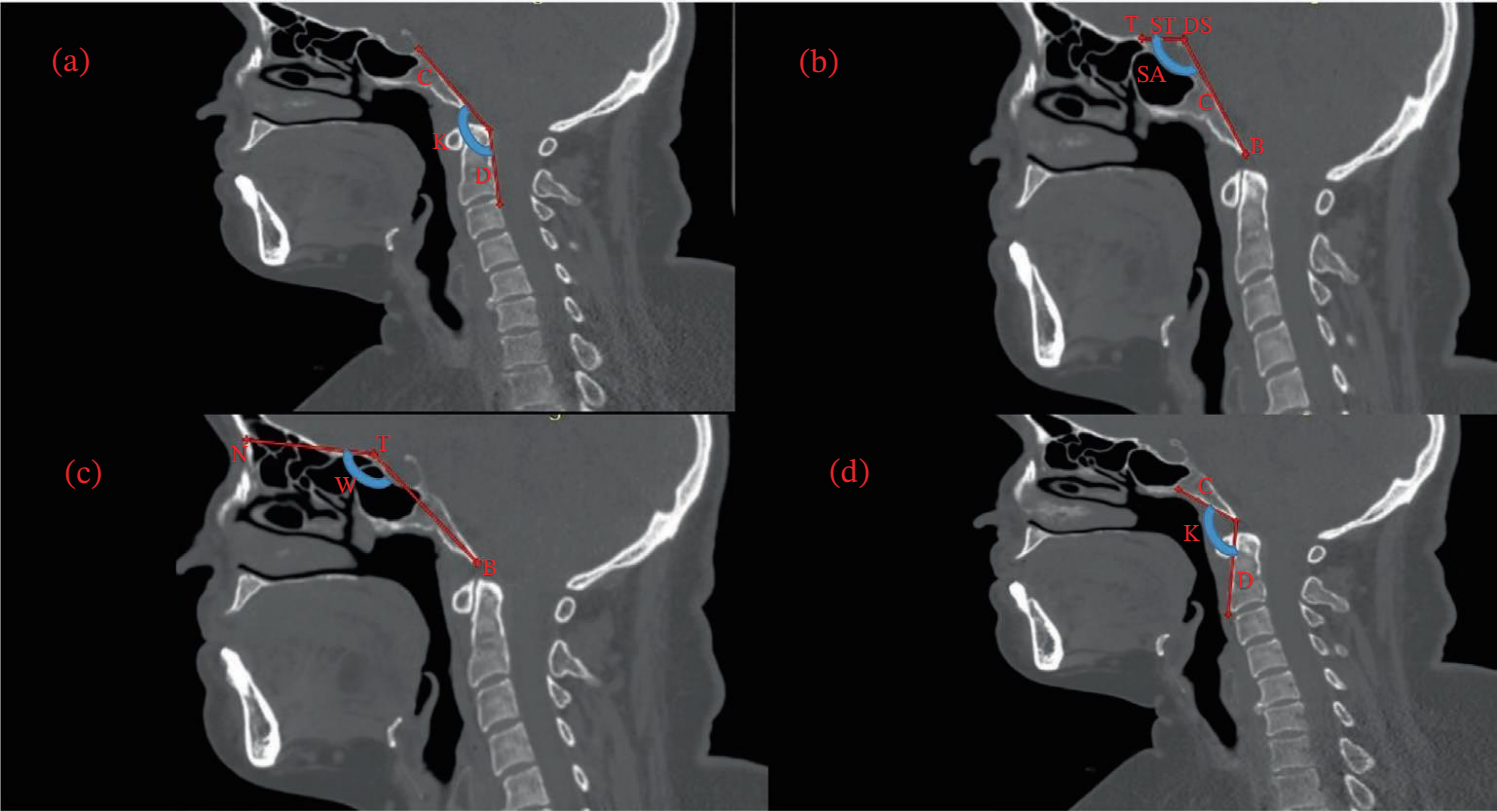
Midsagittal CT angular measurements of craniovertebral relationships.(a) Wachenheim clivus‐canal angle (K°, blue line) formed by lines along the posterior clivus (C) and posterior cervical canal at the dens (D), assessing brainstem compression risk. (b) Sphenoid angle (SA°, blue line) measured between the sphenoid base (ST), dorsum sellae (DS), and clivus (C), evaluating skull base flattening.(c) Welcher basal angle (W°, blue line) formed by basion (B)‐tuberculum sellae (T) and nasion (N)‐tuberculum sellae (T) lines, indicating platybasia when >140°.(d) CTA (K°, blue line) between anterior clivus (C) and dens axis (D), reflecting head‐neck alignment.
